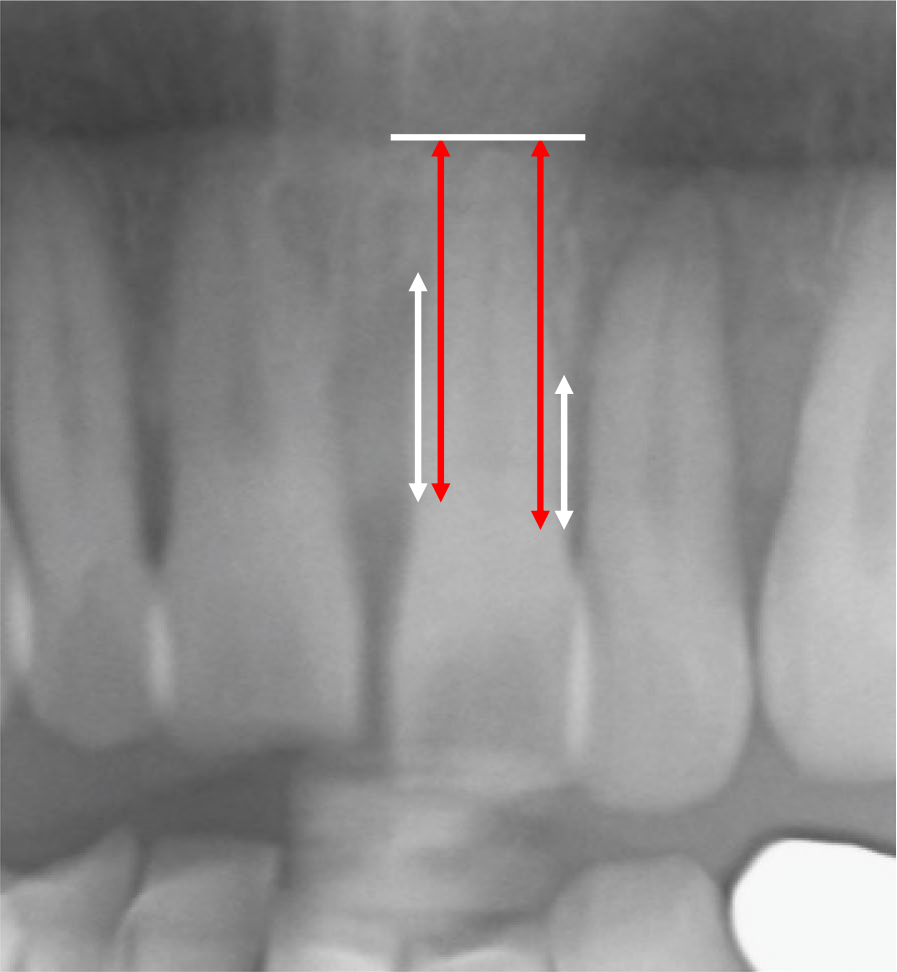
Methods for assessing periodontal bone defects caused by periodontitis on panoramic radiographs. A cropped panoramic tomograph is shown. The alveolar bone defect caused by periodontitis is assessed on a panoramic radiograph by measuring the distance between the cement-enamel junction and the alveolar crest (arrows) and between the cement-enamel junction and the root apex (red arrows) at mesial and distal sites on each tooth. The alveolar bone defect caused by periodontitis of each subject is calculated as the average of the cement-enamel junction-alveolar crest/cement-enamel junction-apex of all teeth
